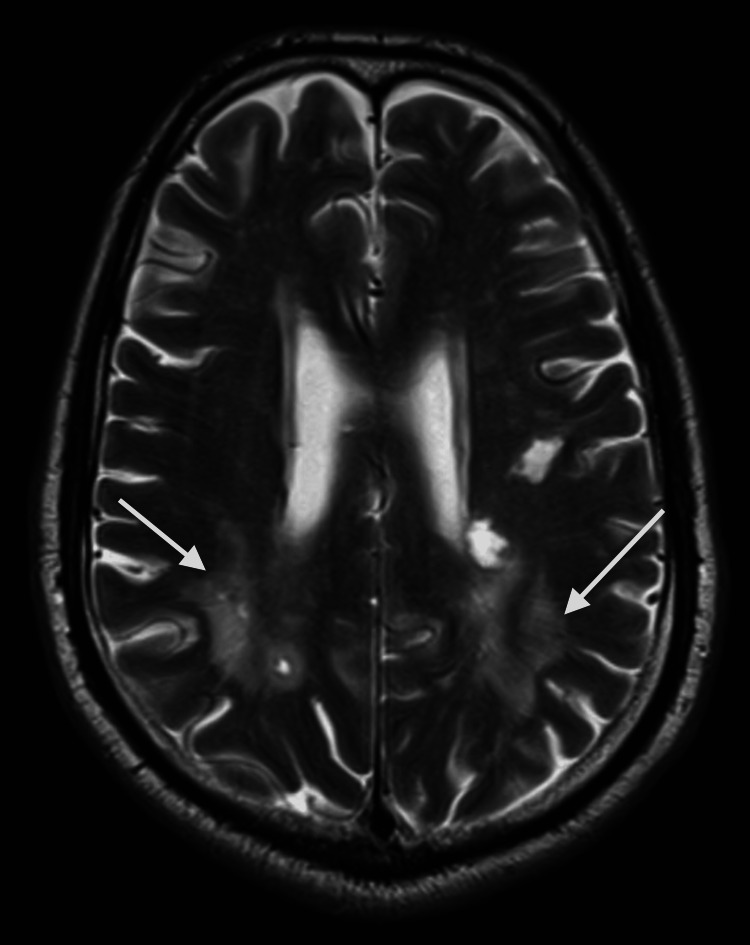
Brain MRI, T2, axial view
